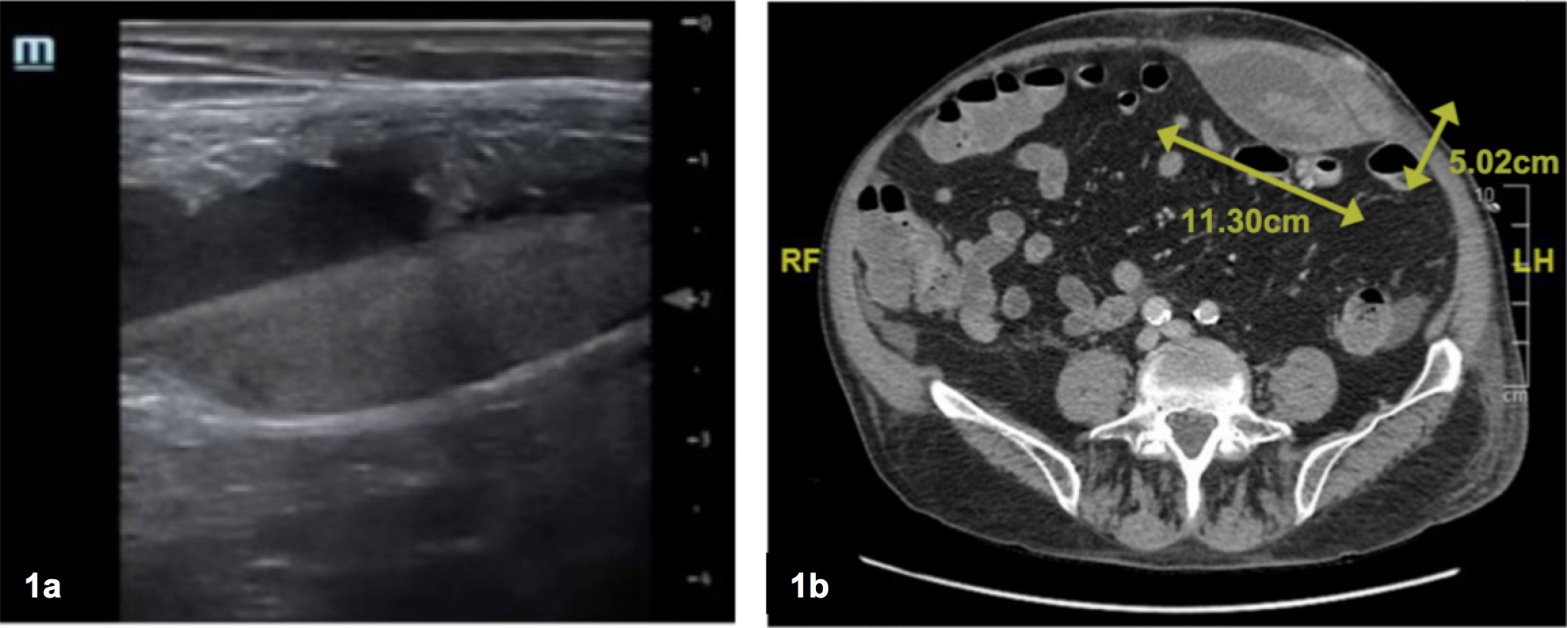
a) Point of Care Ultrasound of Left Lower Abdominal Quadrant mass showing the hematocrit sign. b) CT abdomen and pelvis showing a 11x11x5cm rectus sheath hematoma.
